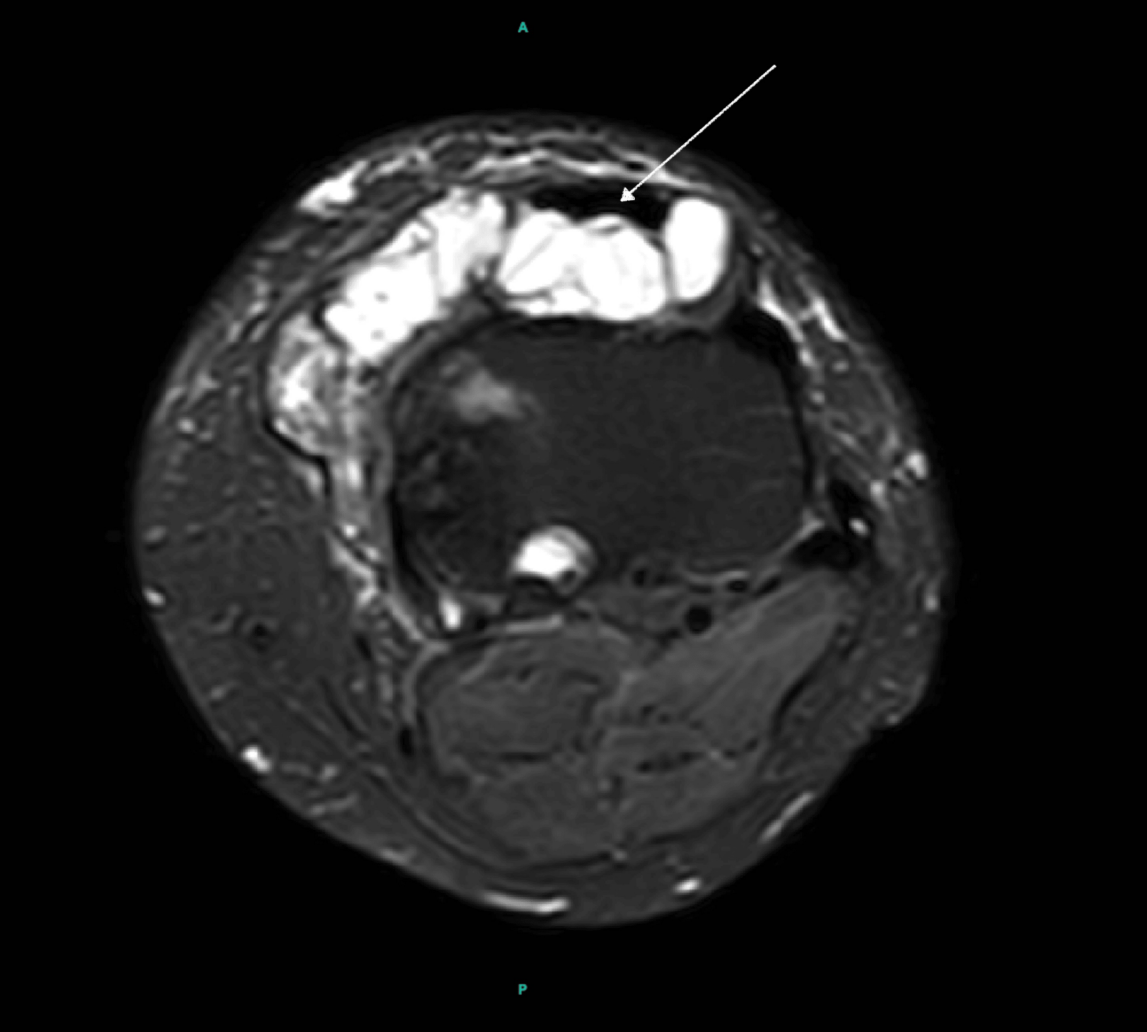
MRI demonstrating axial view of the left knee showing cyst extension into the medial joint capsule (white arrow).
